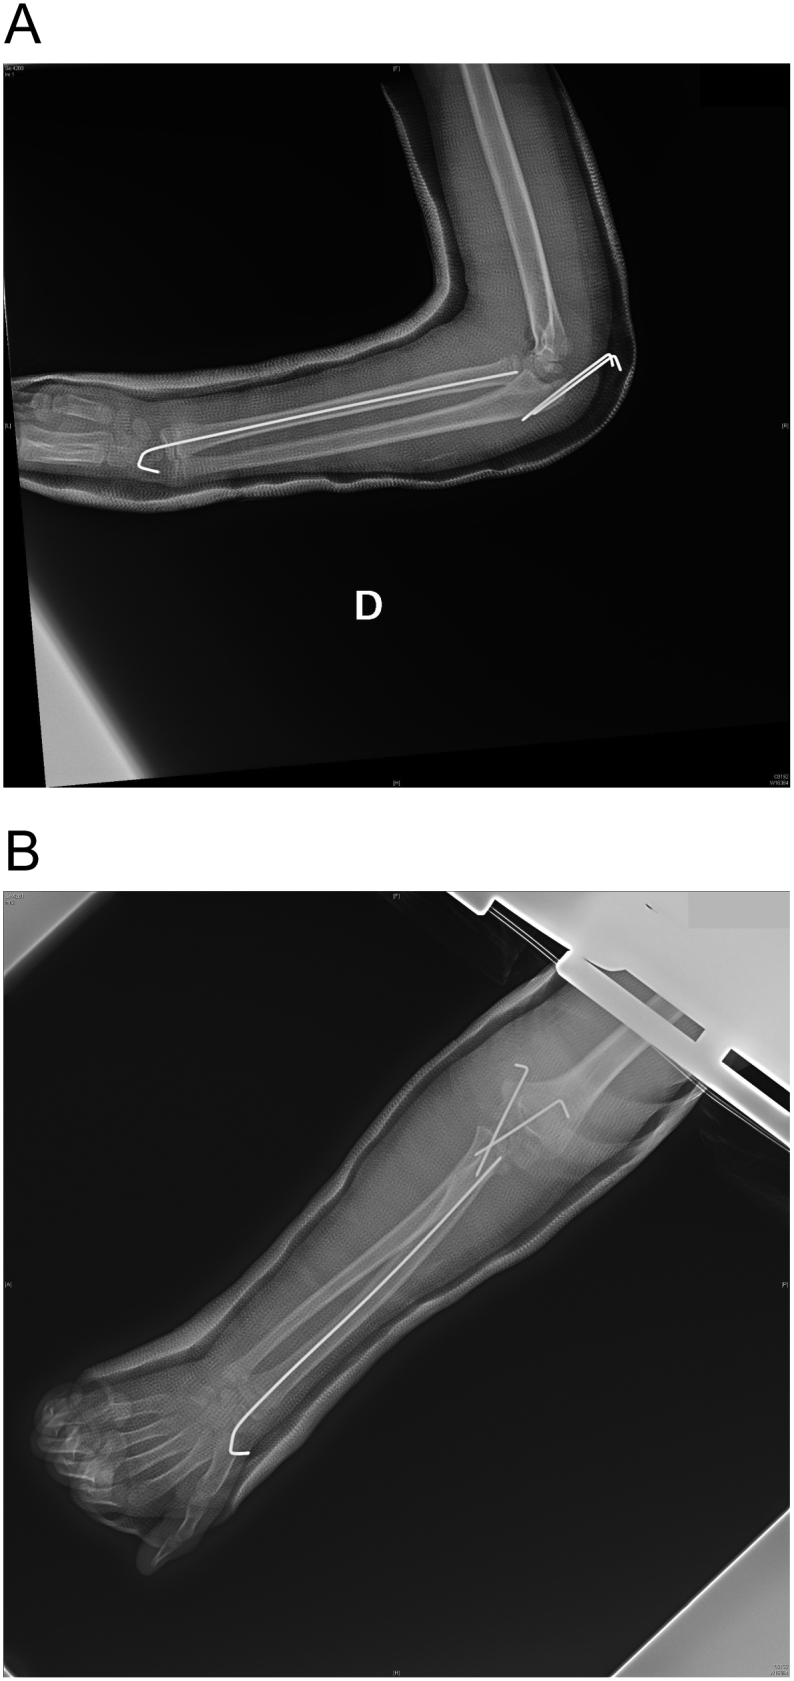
a–b: The post-operative elbow X-ray shows the olecranon fractures fixed with two crossing 1.5 mm K-wires and the angulated radial neck fracture fixed with a retrograde 1.5 mm K-wire by S.E.R.I. technique.
